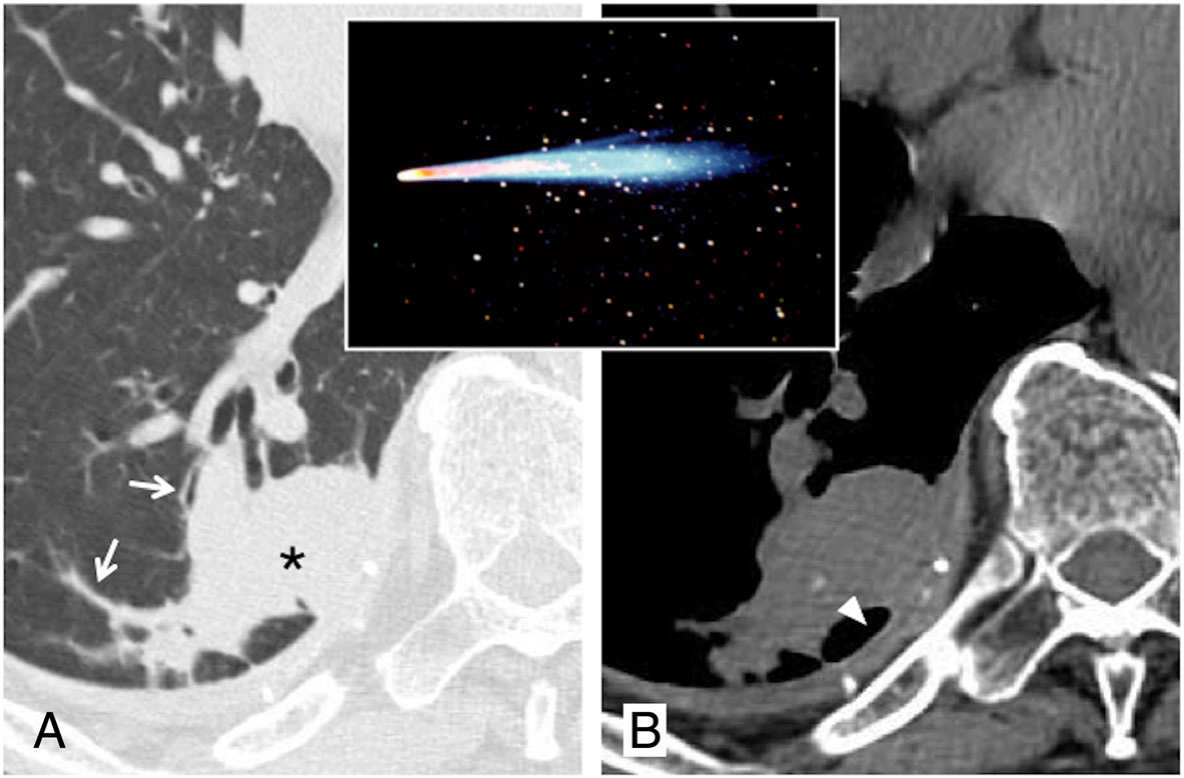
Comet tail sign. Axial CT image (a) shows a round consolidation (black asterisk) and stretched vessel and bronchus (white arrows): these combinations—reproducing a “comet tail” at the border of a round lesion—suggest the presence of round atelectasis. On axial CT image (b, mediastinal window), a dorsal pleural plaque with calcification is also appreciable (white arrowhead)
